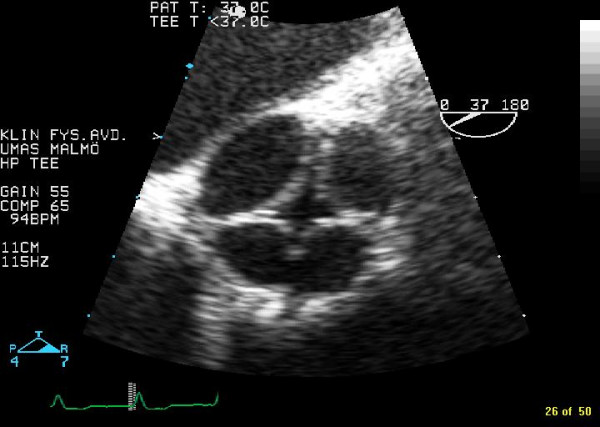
Transoesophageal images, in diastole, of a quadricuspid aortic valve with three cusps of equal size and one larger cusp.
